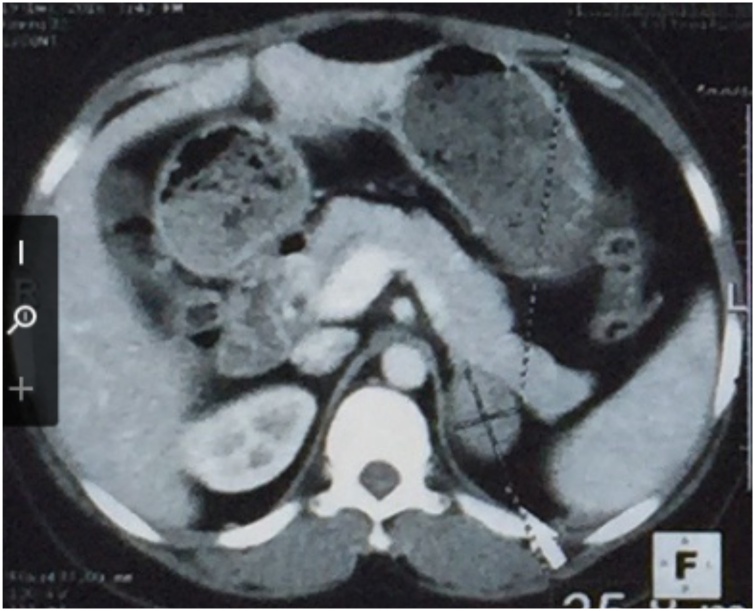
CT scan showing left adrenal mass (case 7).
